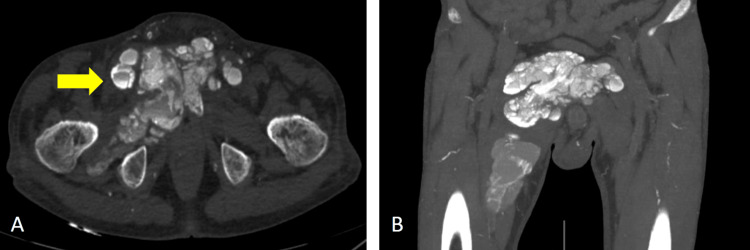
Axial and coronal plain CT scan of the pelvic region Multilobulated calcified mass with areas demonstrating sedimentation (arrow) measuring 12x11.6x9 cm (CCWAP). CT: computed tomography
